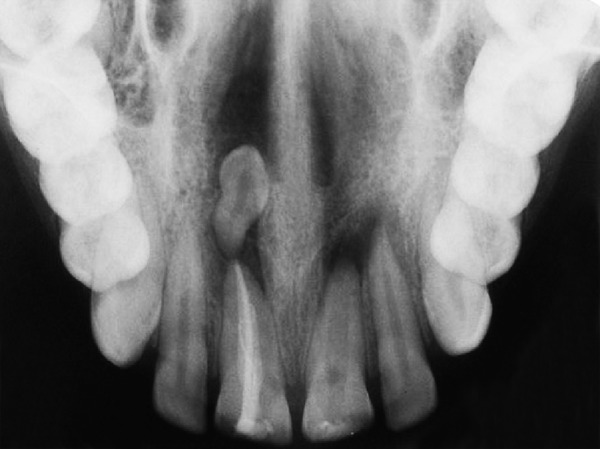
Occlusal radiograph revealing endodontically treated 11
